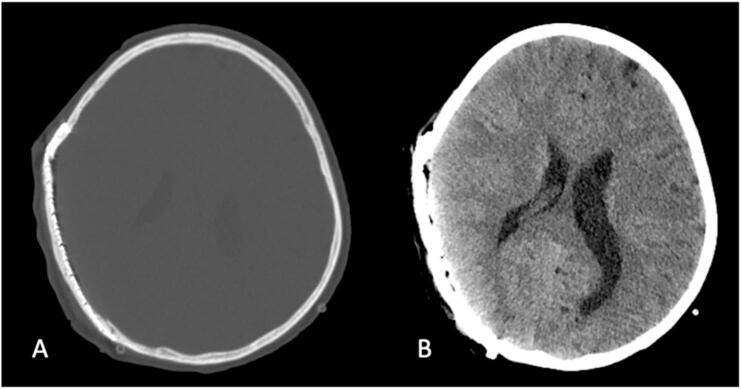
Postoperative computed tomography showing complete mass removal with titanium mesh cranioplasty, (A) bone window and (B) brain window.
